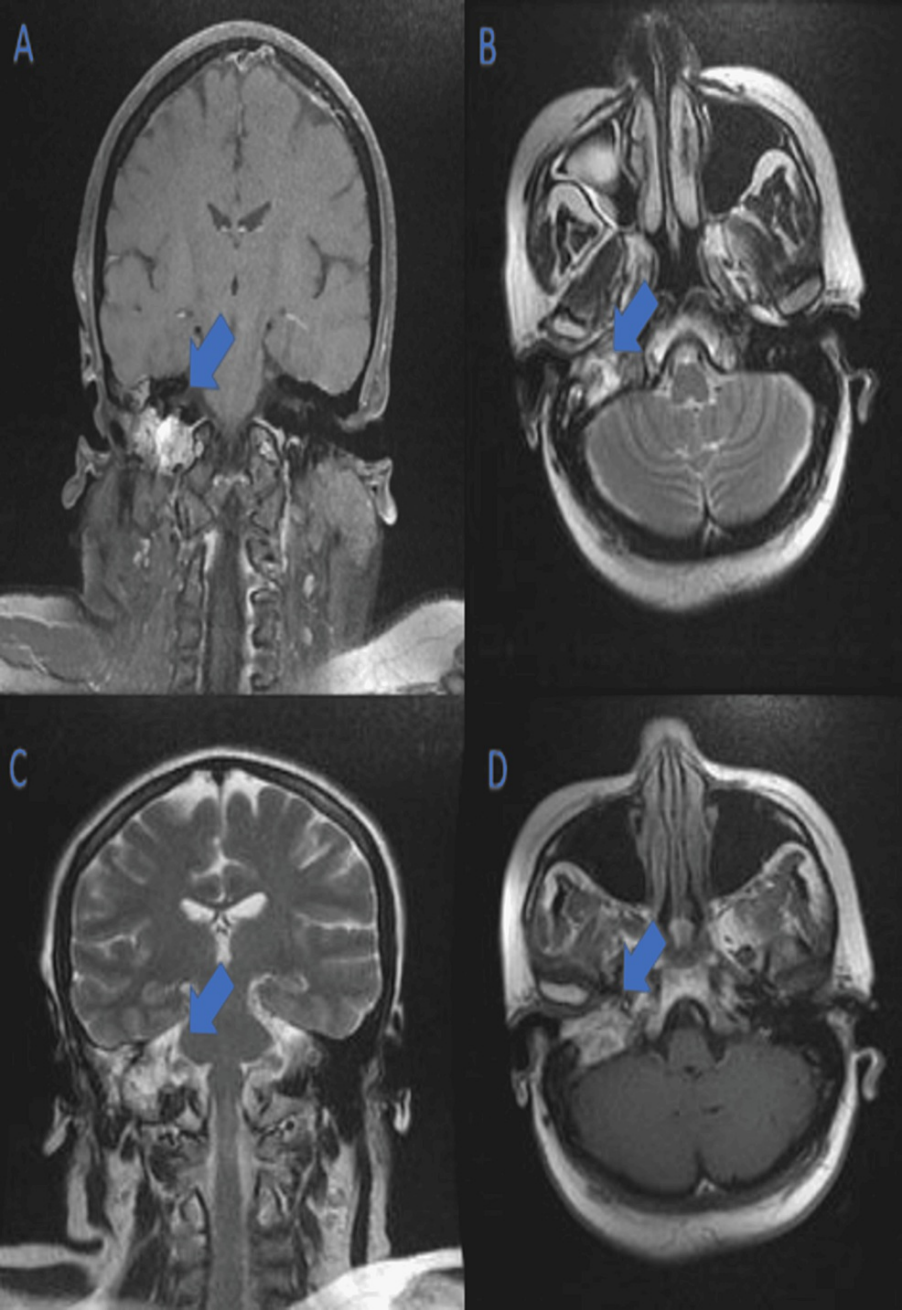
Cerebral MRI with angiographic sequences. (A) Post-contrast T1-weighted coronal section; (B) axial T2 weighted section; (C) coronal T2 section, and (D) post-contrast T1 weighted axial. A-D images show a lesion centered on the jugulo-tympanic axis in hypointense T1 and hyperintense. T2 post-contrast images show intense enhancement of the lesion.
